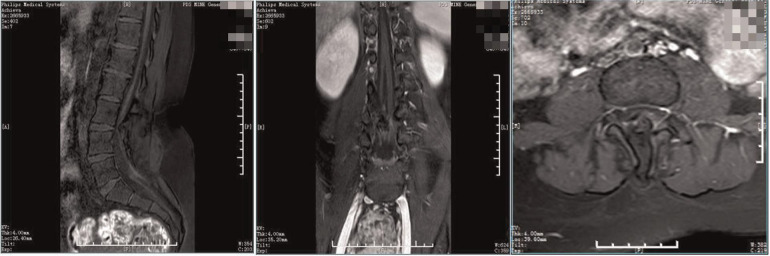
Preoperative enhanced MRI. The spine was diastematomyelia, with tethered spinal cord and terminal filament lipoma, infusion of the lamina at L3-5 segments, and local lamina thinning.
